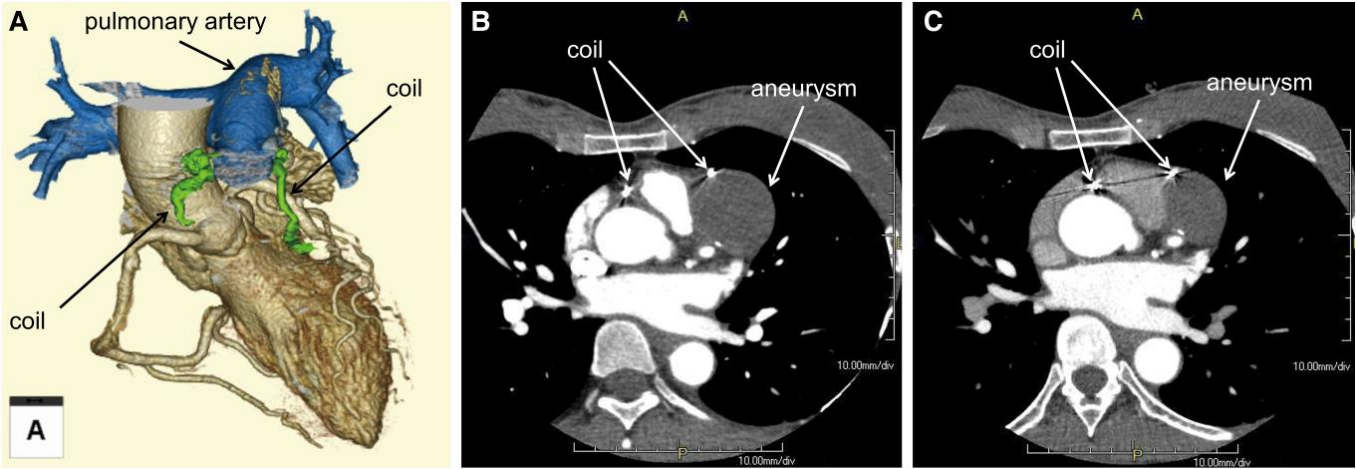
(*A*) Three-dimensional reconstructed contrast-enhanced computed tomography (CT) image after coil embolization. (*B* and *C*) Serial contrast-enhanced CT images, (*B*) 2 days after the procedure, and (*C*) 1 year later.
